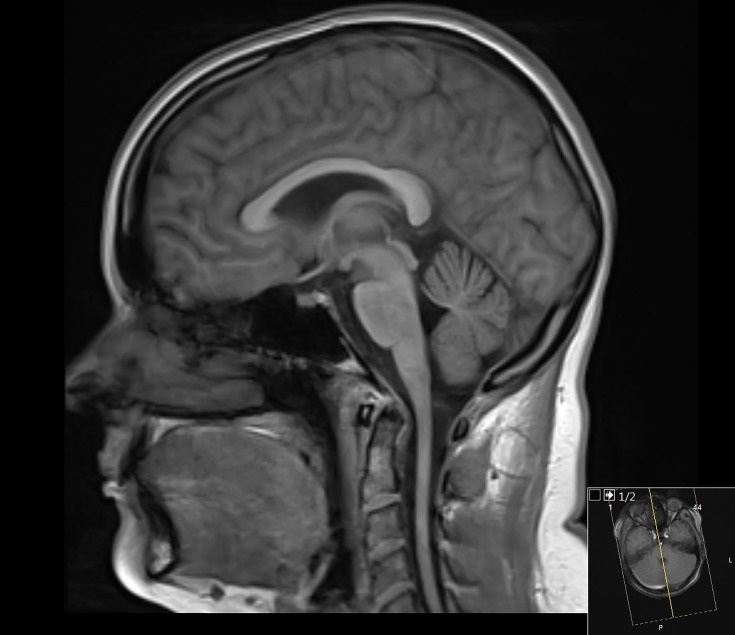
Sagittal T1-weighted MRI of the brain and upper cervical cord in a 26-year-old woman with ataxia. There is relative preservation of the brainstem and cerebellum, but thinning of upper cervical cord. (Friedreich’s ataxia (FRDA) was confirmed in her genetically.)
